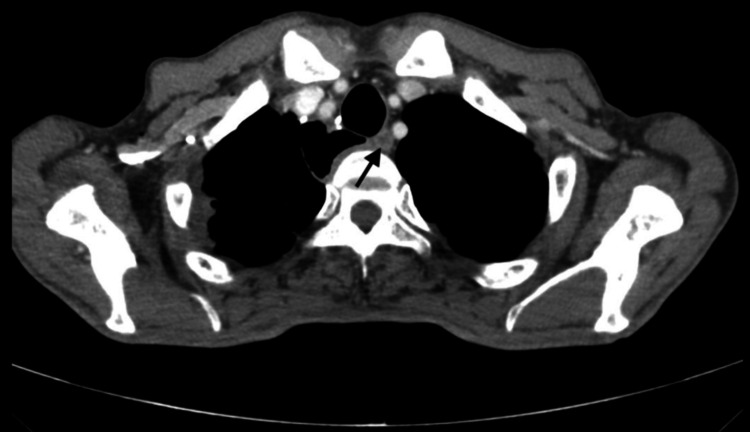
Axial CT image demonstrating a recurrent mediastinal lymph node (106recL) (arrow) CT: computed tomography
